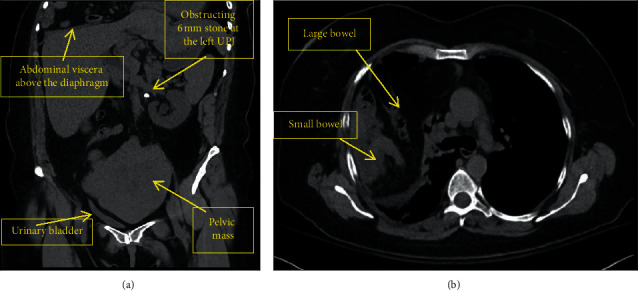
Axial (a) and coronal (b) views of CT (computed tomography) thorax, abdomen, and pelvis which revealed 6 mm left obstructing ureterolithiasis with hydronephrosis, as well as incidentally noted large 13 cm heterogeneous mass within the pelvis resulting in distortion of local structures. Additionally visualized was a large Morgagni hernia containing a large majority of the small bowel, the transverse colon, a portion of the descending colon, and a portion of the stomach with significant adjacent atelectasis of right lower lung lobe.
